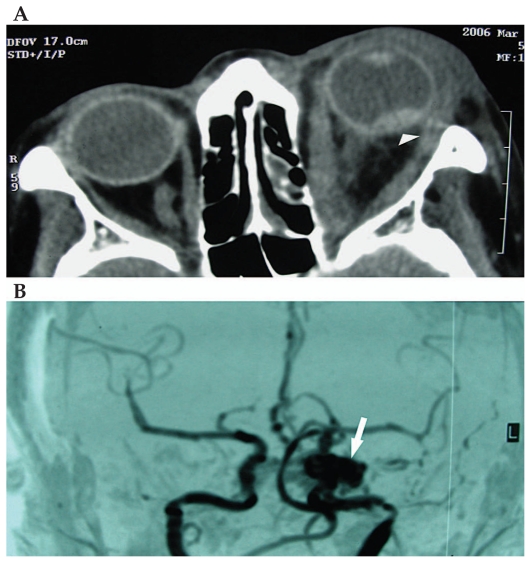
(A) Axial computed tomography scan of the orbit: the left eye is severely proptotic, extraocular muscles are enlarged and an intraocular hemorrhage is noted (arrowhead). (B) Magnetic resonance angiographic view of a spontaneous high flow carotid-cavernous fistula on the left side (arrow).
